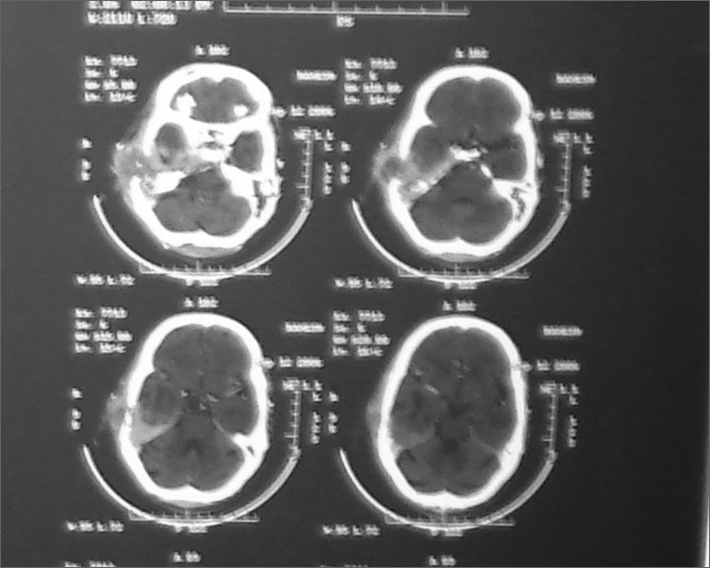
CT scan temporal bone showing the local destruction with intracranial extension
